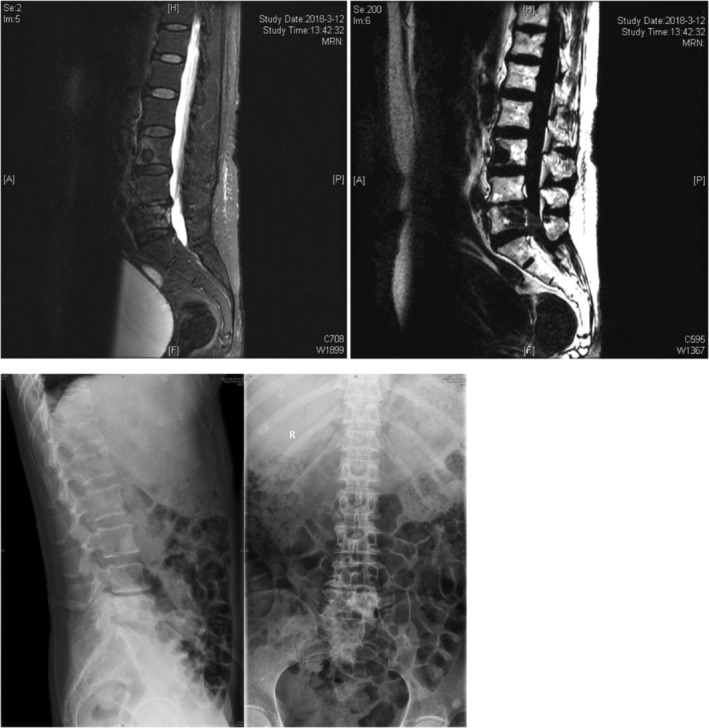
Preoperative MRI and X‐ray scan.
